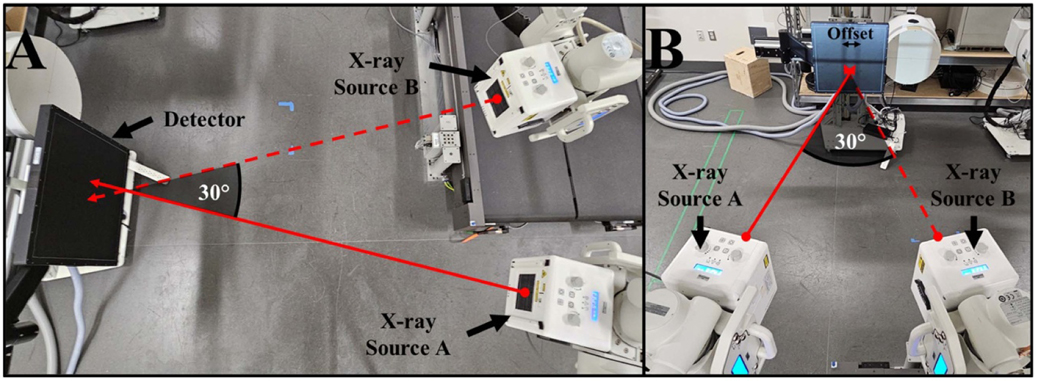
The monoplane stereoscopic videoradiography (MSV) configuration. (A) X-ray sources A and B are obliquely aligned to a single detector. This enables the participant to be positioned adjacent to the detector, minimizing the object-to-image distance. (B) X-ray sources A and B were aligned obliquely to a single detector with an inter-beam angle of 30°. The projections onto the detector were vertically centered and horizontally offset.
